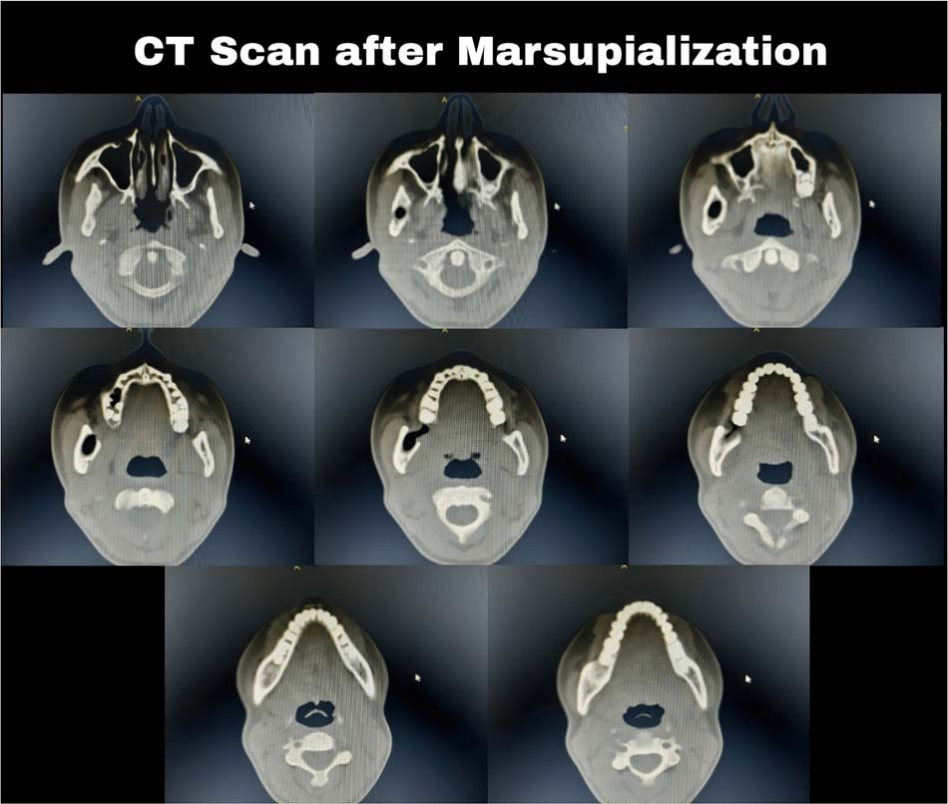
Computed tomography after 12 months showed a bone regeneration in the lesion’s area and the cystic lesion has become completely surrounded by thick bony plates.
